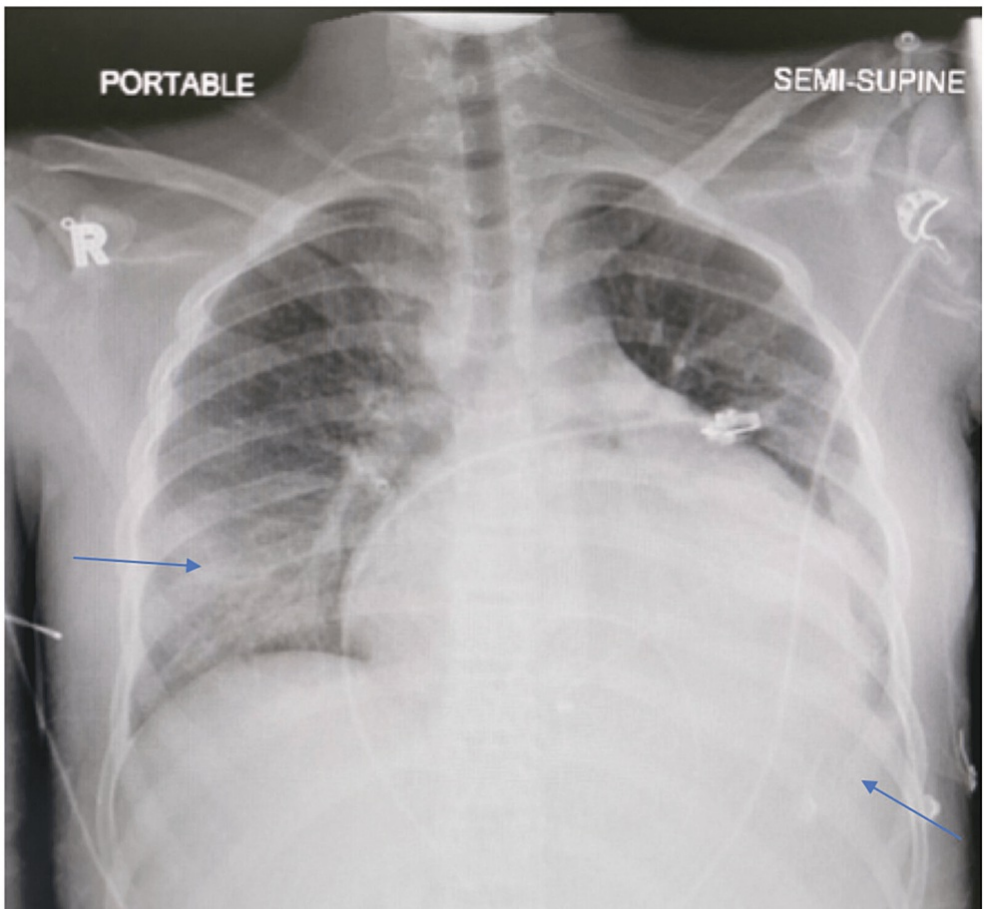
Chest X-ray in Case 3 showed bilateral lower lobe atelectasis with left-sided pleural effusion.
